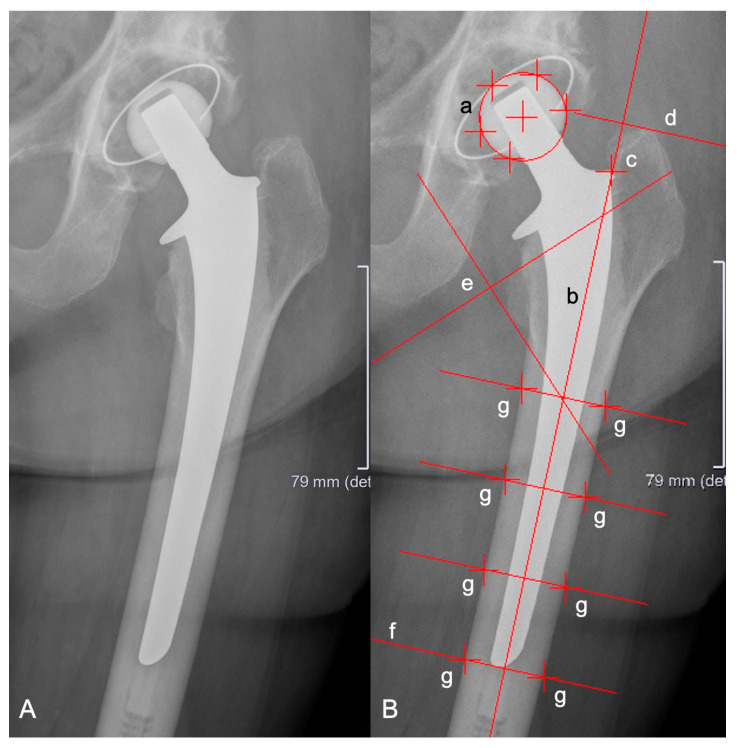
Anterior-to-posterior X-rays showing a cemented SP2 stem (A) and with EBRA-FCA references (B): (a) head points, (b) stem axis, (c) stem shoulder, (d) major trochanter line, (e) minor trochanter lines, (f) tip-of-stem line, and (g) points at femoral bone contour.
